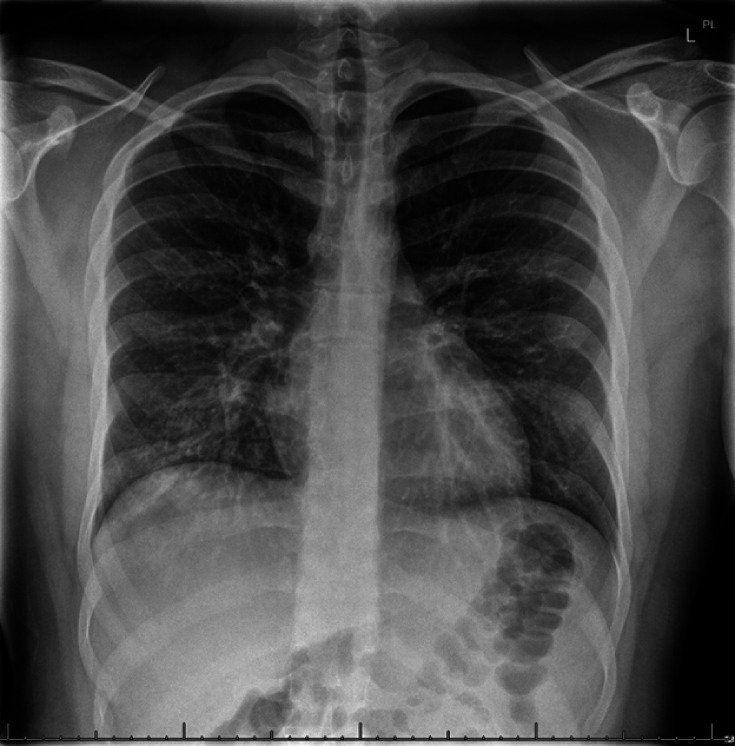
Chest X-ray taken on admission, demonstrating right lower lobe consolidation consistent with CAP.
